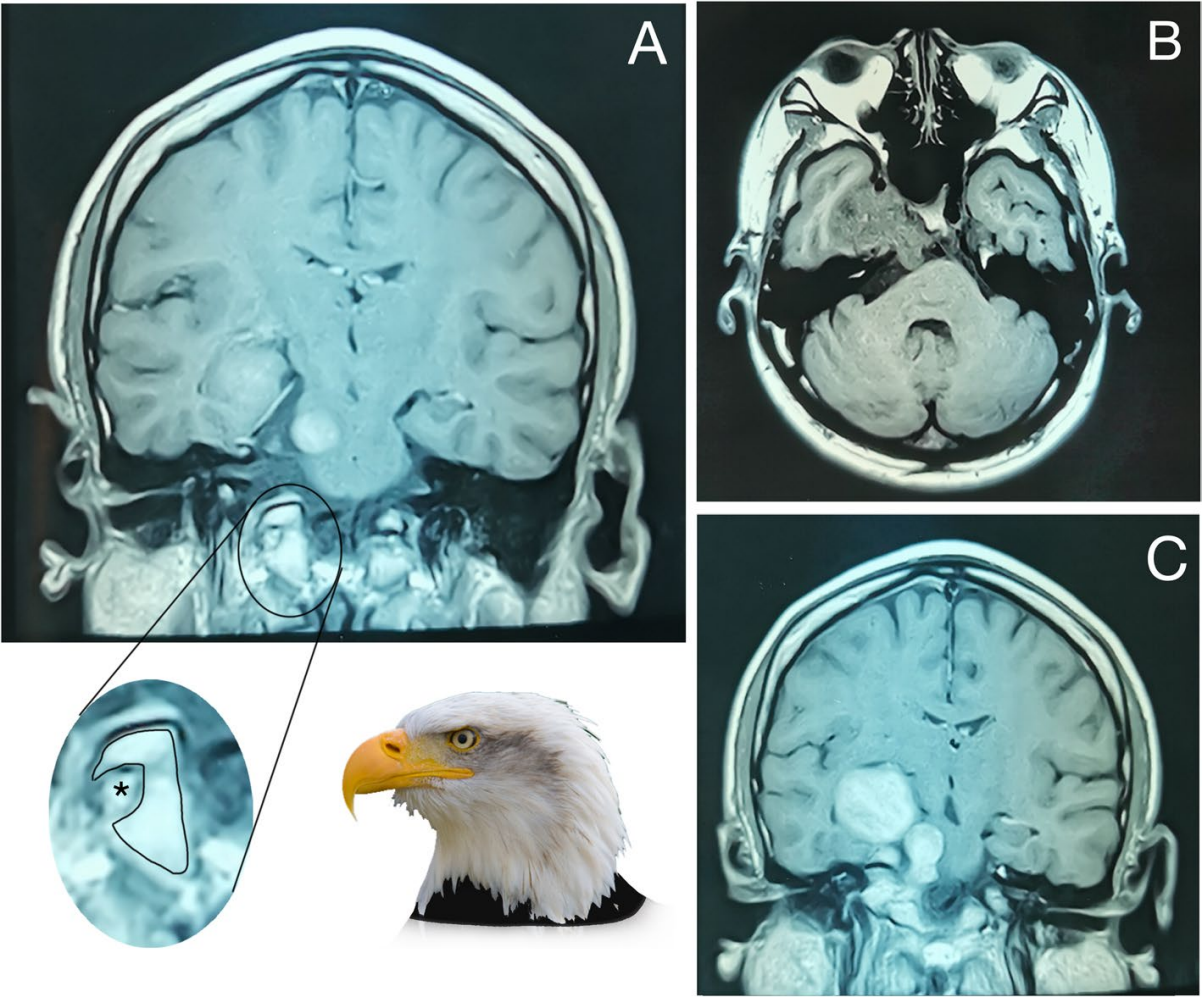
A Coronal view of the MRI brain shows residual tumour at the right basiocciput of the clivus. Enlarged photo shows tumour involving the right hypoglossal canal (in asterisk, *) Hypoglossal canal surrounded by jugular tubercle and occipital condyle resembles eagle head. (Eagle head image from Wikimedia Commons). B Axial view of MRI brain shows right clival mass at prepontine cistern causing compression the pons. C Residual clival chondrosarcoma extending to the right side sella compressing and displacing pituitary gland to the left
